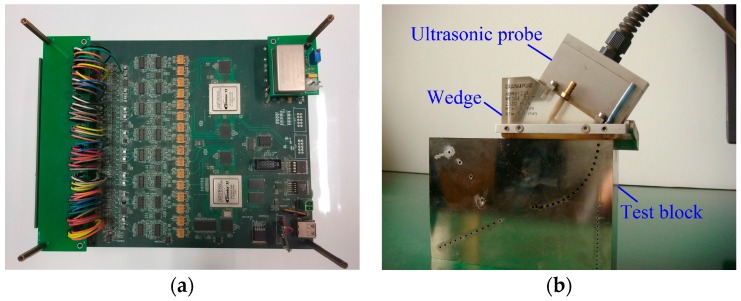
The ultrasonic phased array detection system. (a) Circuit board of ultrasonic transmitting and defect echo receiving; (b) Ultrasonic probe and standard test block.
